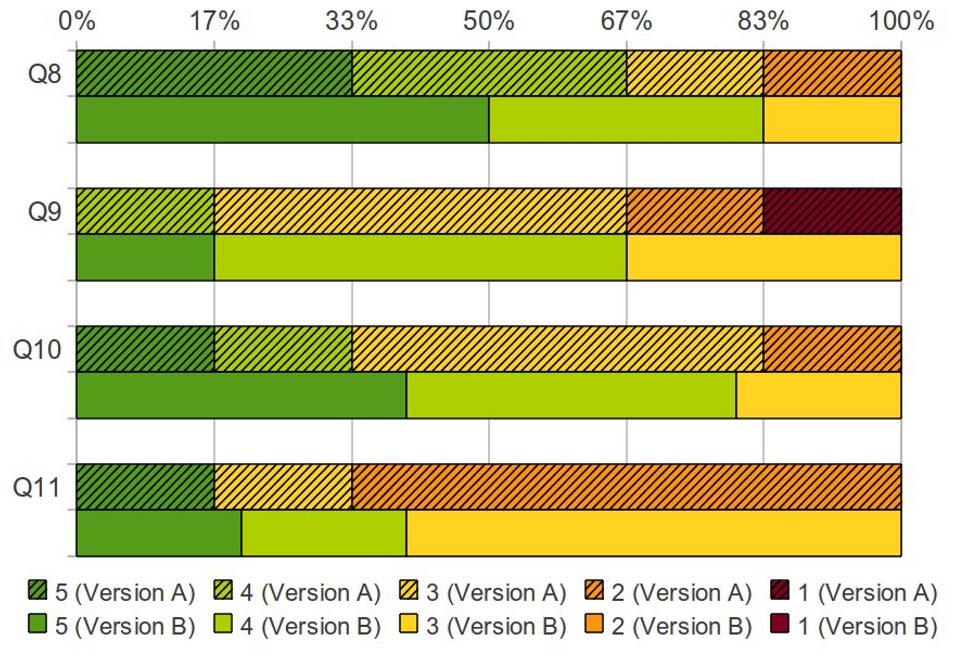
Score distribution on how these models, with/without PET, changed their understanding of the tumor. Percentages of surgeons who assigned a particular score for both Version A (solid colors with hatch marks) and Version B (solid colors) [Q8: By simply viewing the image(s) in the 3D model, I get a better understanding of the tumor and its relationship to the surrounding organs, Q9: By simply viewing the image(s) in the 3D model, my plan for how to approach this tumor changed (as compared to traditional CT images), Q10: By overlaying the PET data in the 3D model, I get a better understanding of the tumor and its relationship to the surrounding organs, Q11: By overlaying the PET data in the 3D model, my plan for how to approach this tumor changed (as compared to traditional CT images)].
